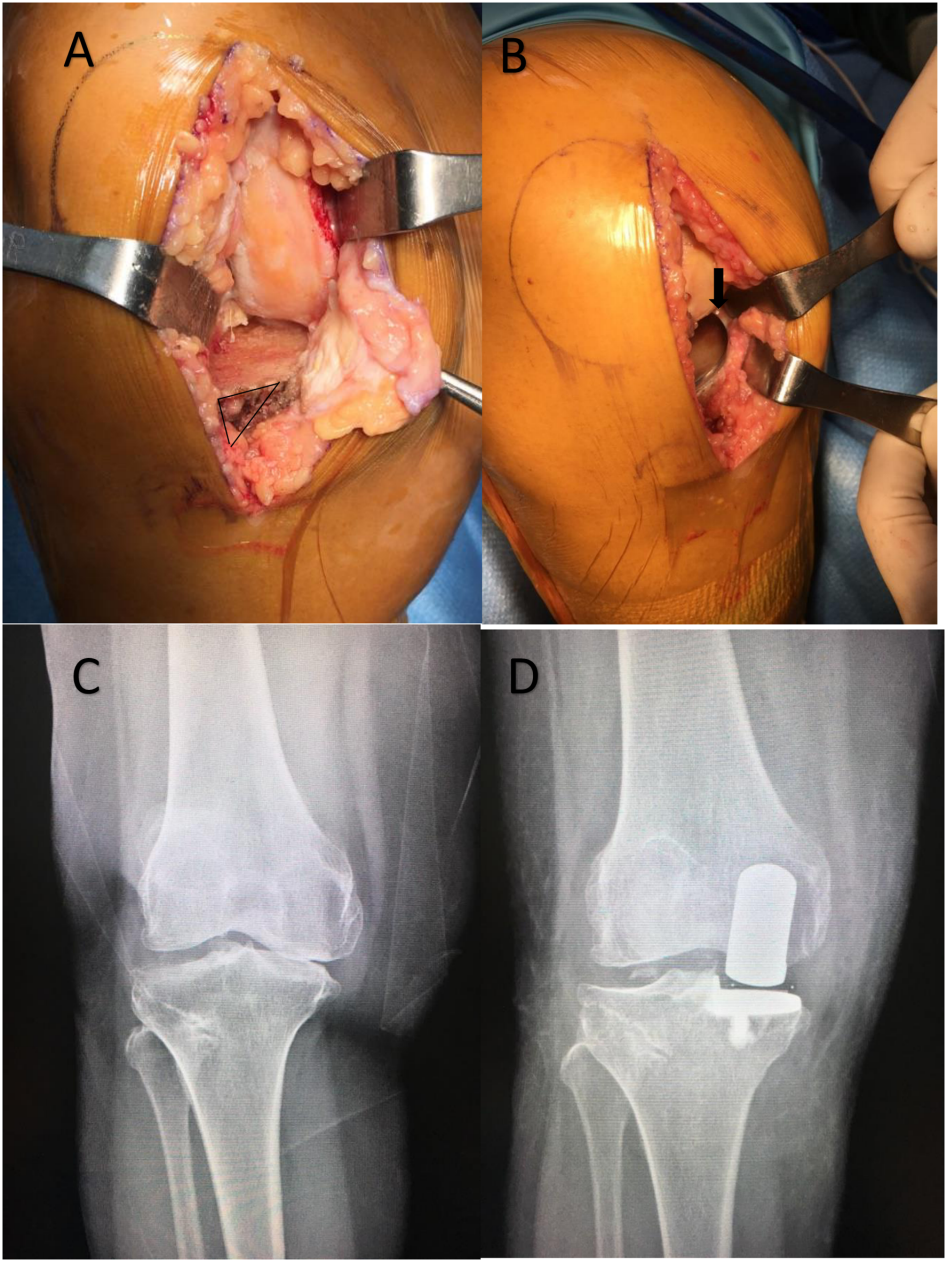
Only the anterior capsule was released when entering the knee joint (triangle shape). Removal of as much of the anteromedial osteophyte was done but always keeping above the insertion of the medial collateral ligament MCL and capsule (A). The anteromedial cortex was identified and used to position of the tibial component to prevent implant overhang. The posteromedial osteophyte (arrow) was difficult to remove without releasing MCL (B). The preoperative radiographic showing the large medial osteophyte (C). The postoperative radiograph (D) showing the residual osteophyte.
